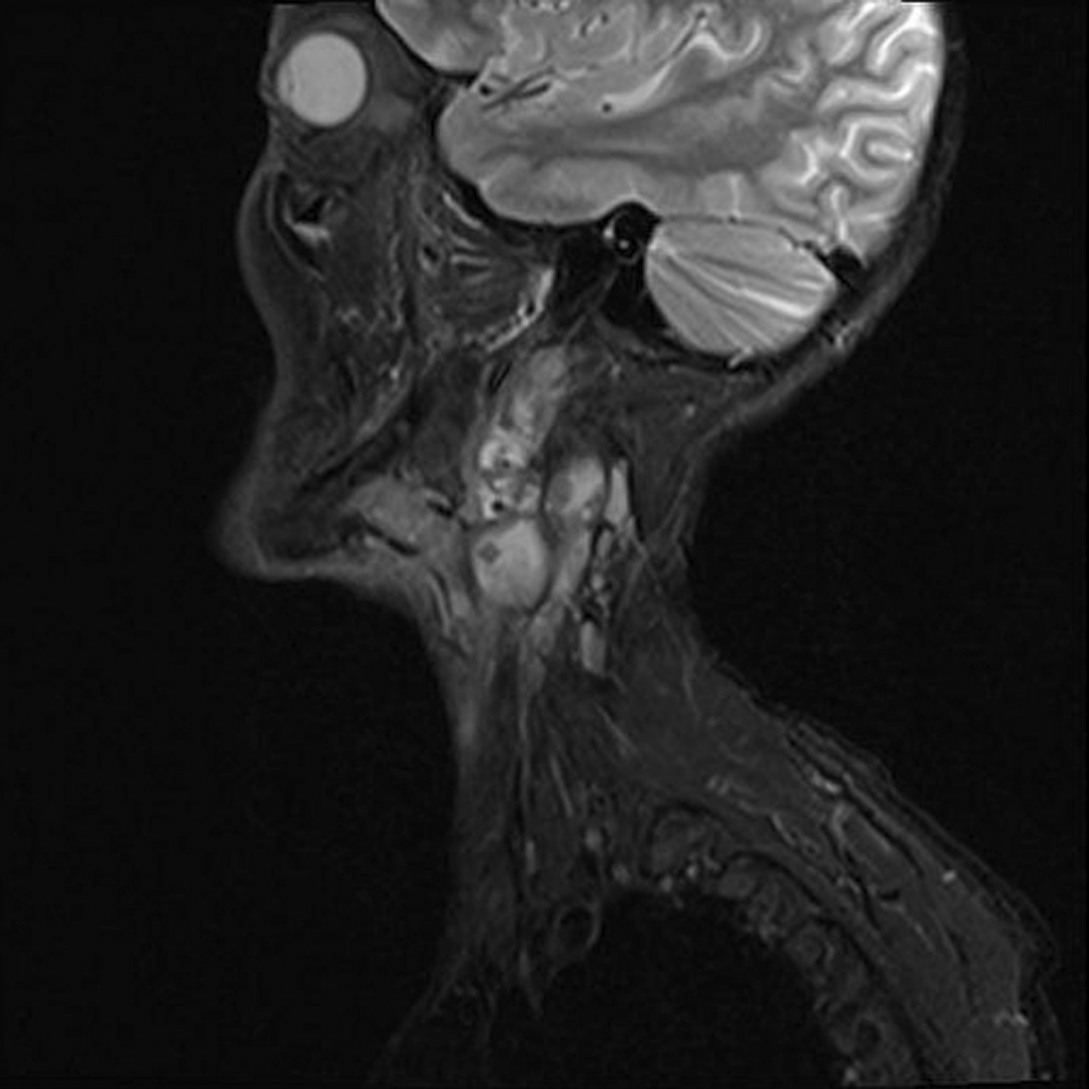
Sagittal T2W brain MRI shows heterogeneous mass legions at IIa, IIb, and III levels on the right side, suggesting metastatic LAP
